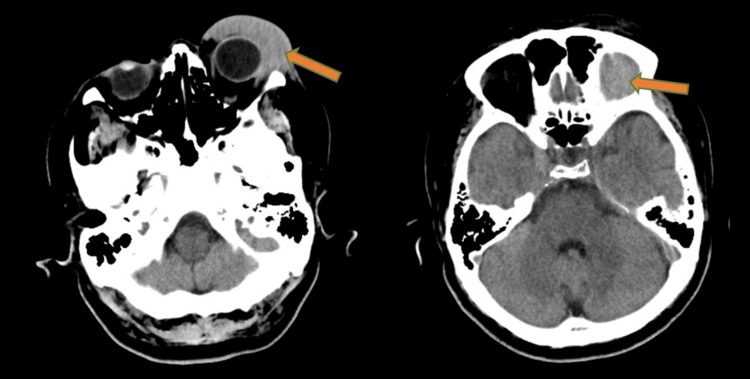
Plain CT orbit; axial cut showing hyperdensity at the left periorbital and superior extraconal regions extending to orbital apex measuring 4.5x3.8x3.2cm (orange arrow)
